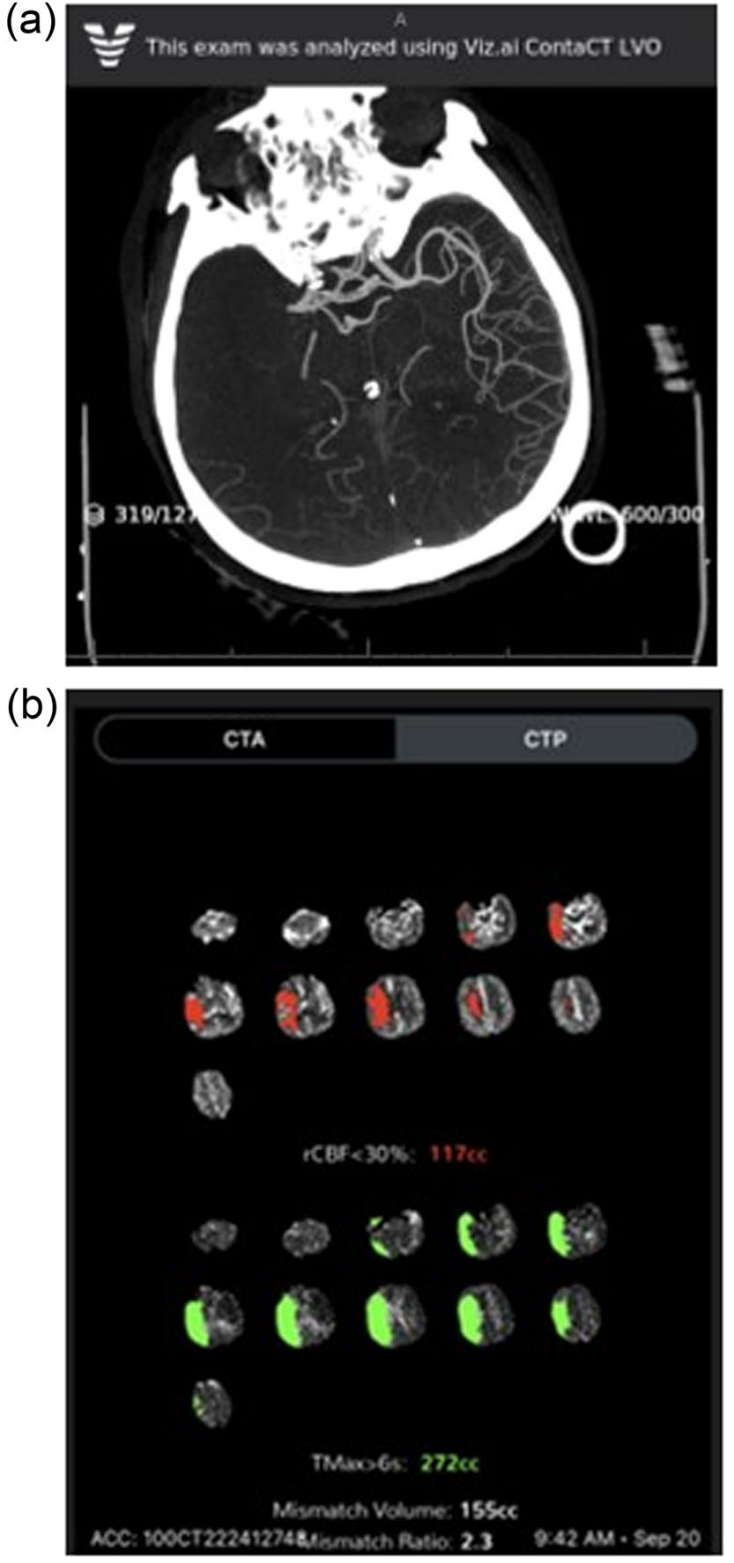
Example of Viz.ai's automated large vessel occlusion detection software and computed tomography perfusion output. (a) Automated output of Viz.ai's large vessel occlusion detection software demonstrating a proximal right middle cerebral artery (MCA)‐M1 occlusion. (b) Automated computed tomography perfusion output for the same patient showing core infarction of 117 cubic centimeters (cc) and penumbra of 155 cc in the right MCA territory.
